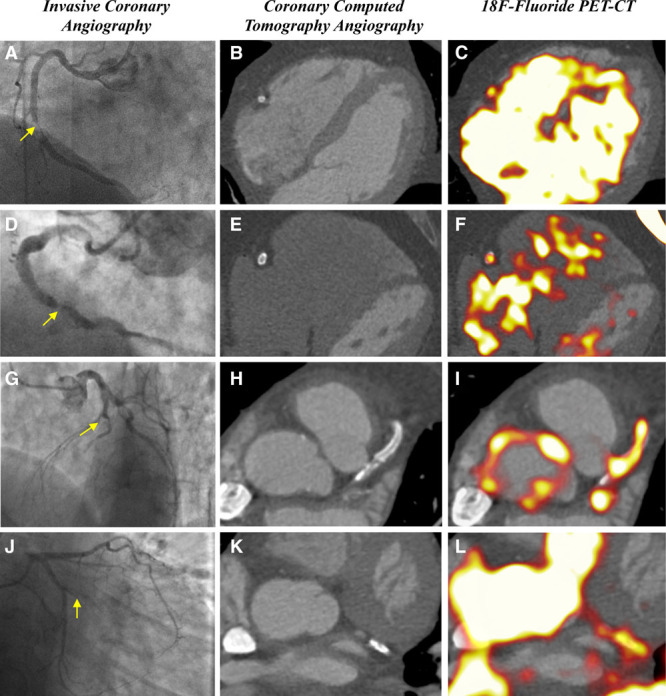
Culprit plaque ^18^F-fluoride activity on positron emission tomography-coronary computed tomography angiography (PET-CT). After acute myocardial infarction, culprit plaque ^18^F-fluoride activity can be measured in the right coronary artery (A–F), left anterior descending artery (G–I), and atrioventricular circumflex artery (J–L).
